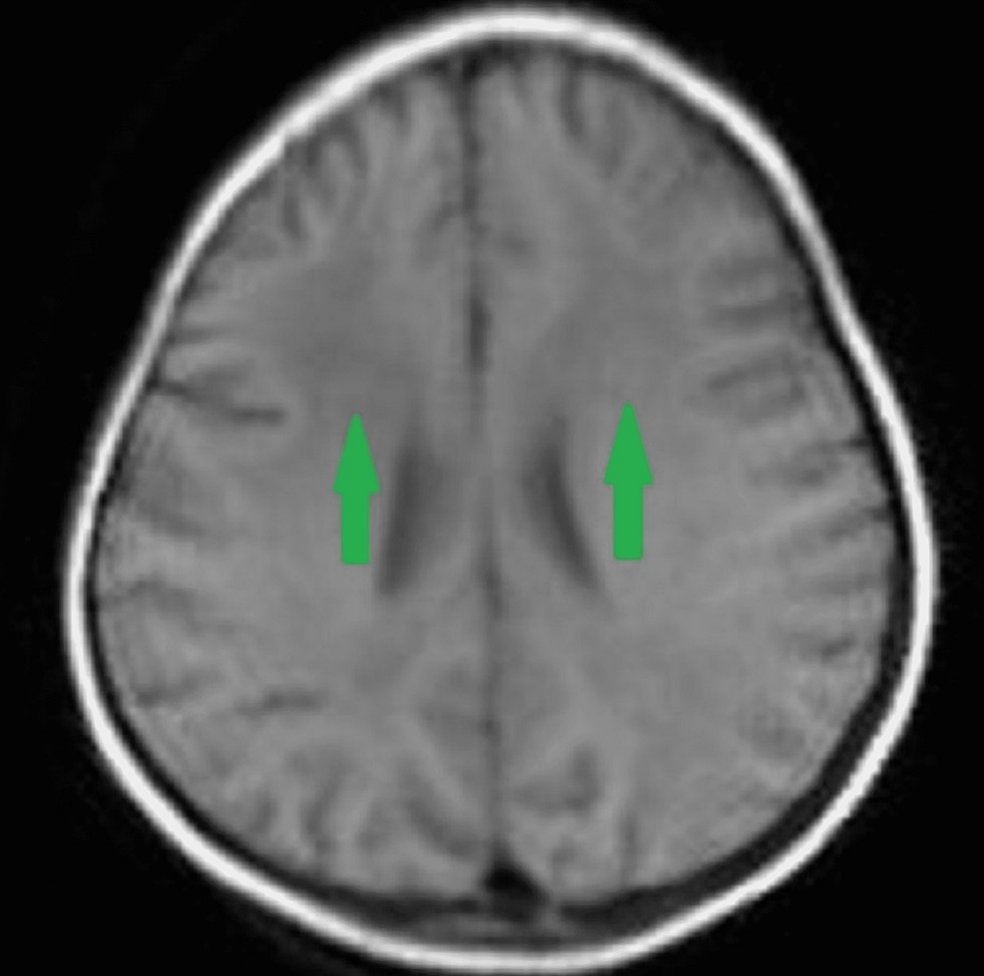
T1W image shows diffuse symmetrical, bilateral hypointensities with preponderance of frontal lobes (green arrows). T1W image: T1-weighted image
